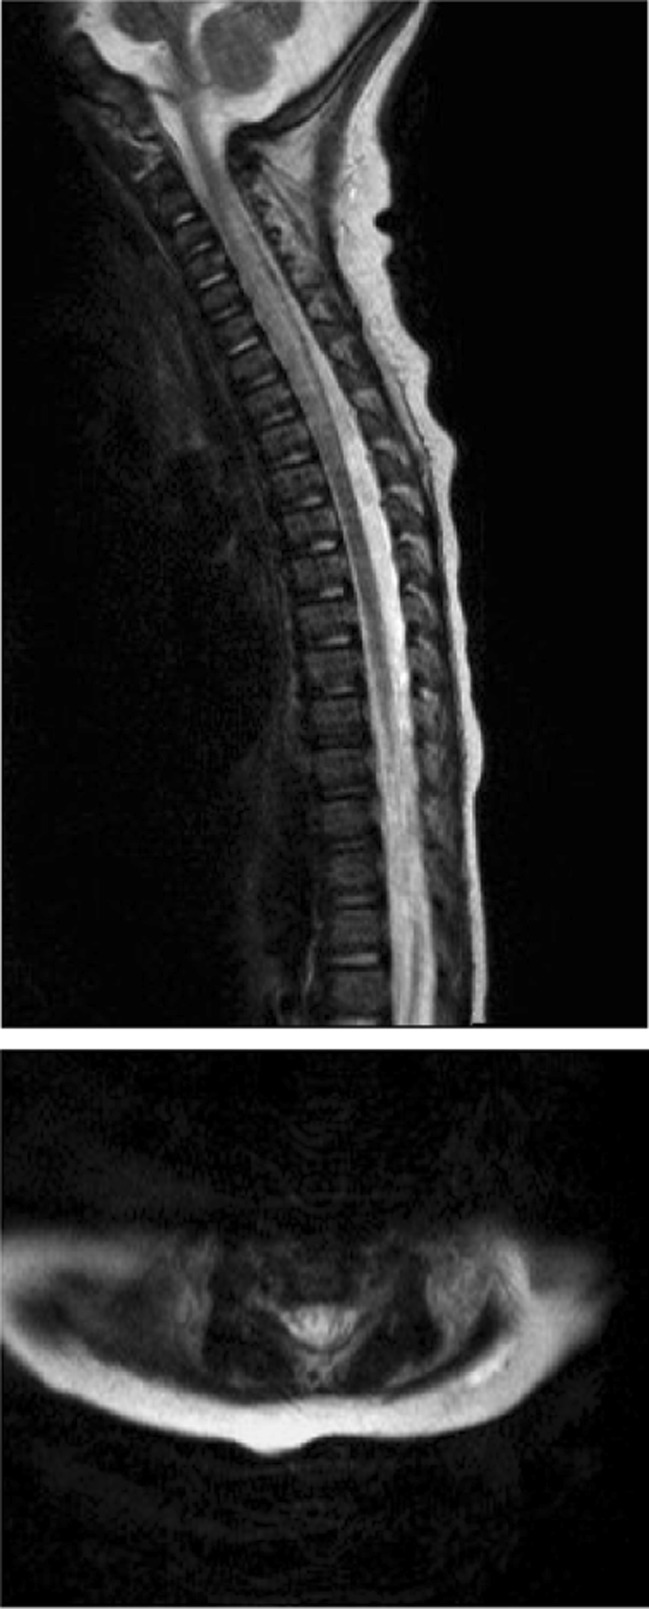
Initial MRI imaging obtained 2 days following surgical intervention. Imaging assessment was significantly degraded by motion artefact; however, the T2 imaging clearly shows abnormal cord expansion and T2 signal change extending between C2/3 and T2
